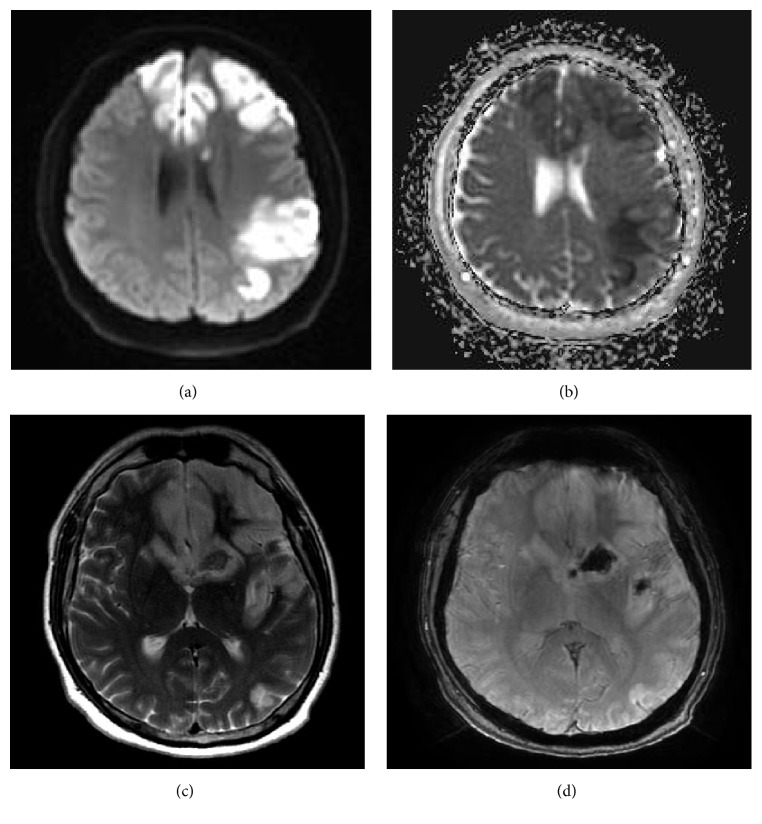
Selected images of MRI of brain. ((a) and (b)) (DWI/ADC) image showing restriction diffusion in bilateral medial frontal lobes and watershed areas in frontal and temporal lobes. (c) T2-weighted image showing hyperintensities in basal ganglia (recurrent artery of Heubner). (d) SWI sequence showing hemorrhagic changes in left basal ganglia and insula.
